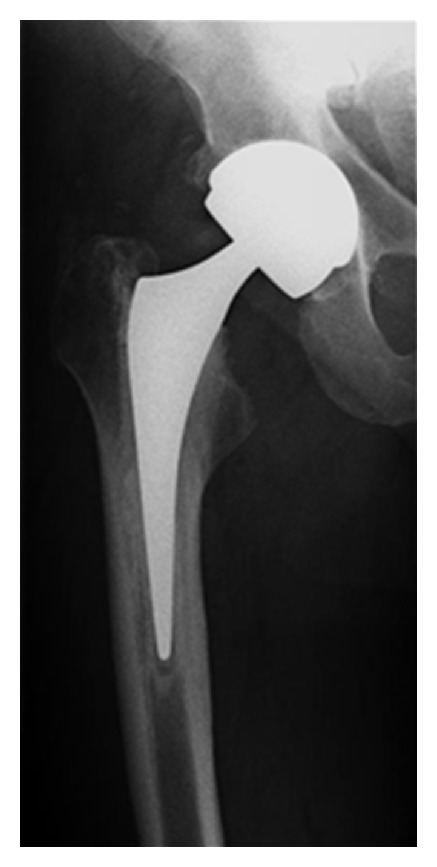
Anteroposterior radiograph following revision surgery using C-stem AMT.
